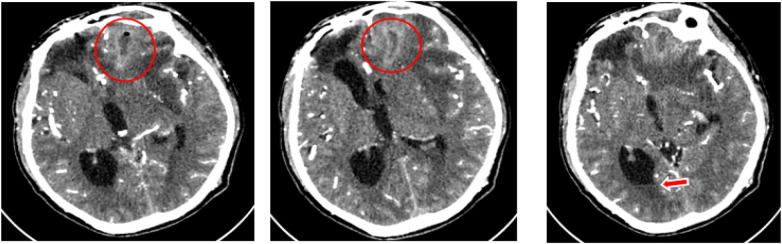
Contrast-enhanced cranial CT: rupture of the brain abscess with significant reduction in volume (red circle); empyema is visualized in the posterior horn of the ventricle (red arrow).
